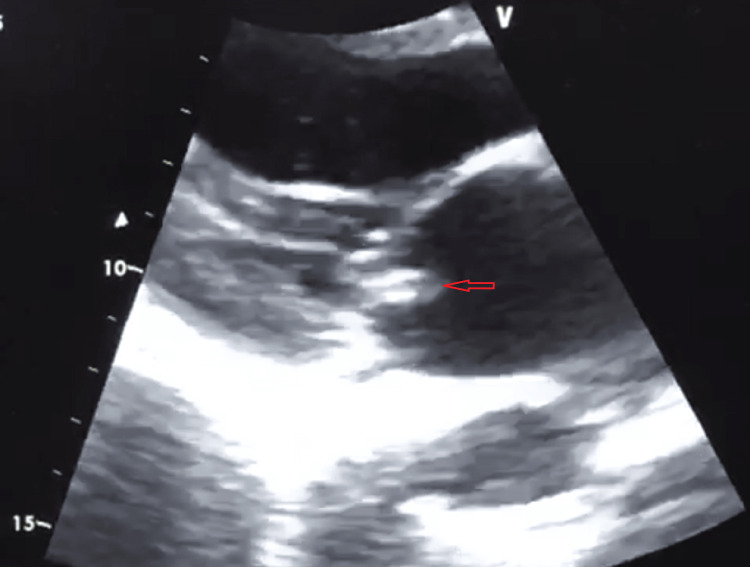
Transthoracic parasternal long-axis view. The image shows the mitral valve apparatus without remodeling or calcifications, with the presence of a hyperechoic mass of irregular consistency, suggestive of vegetation (arrow).
